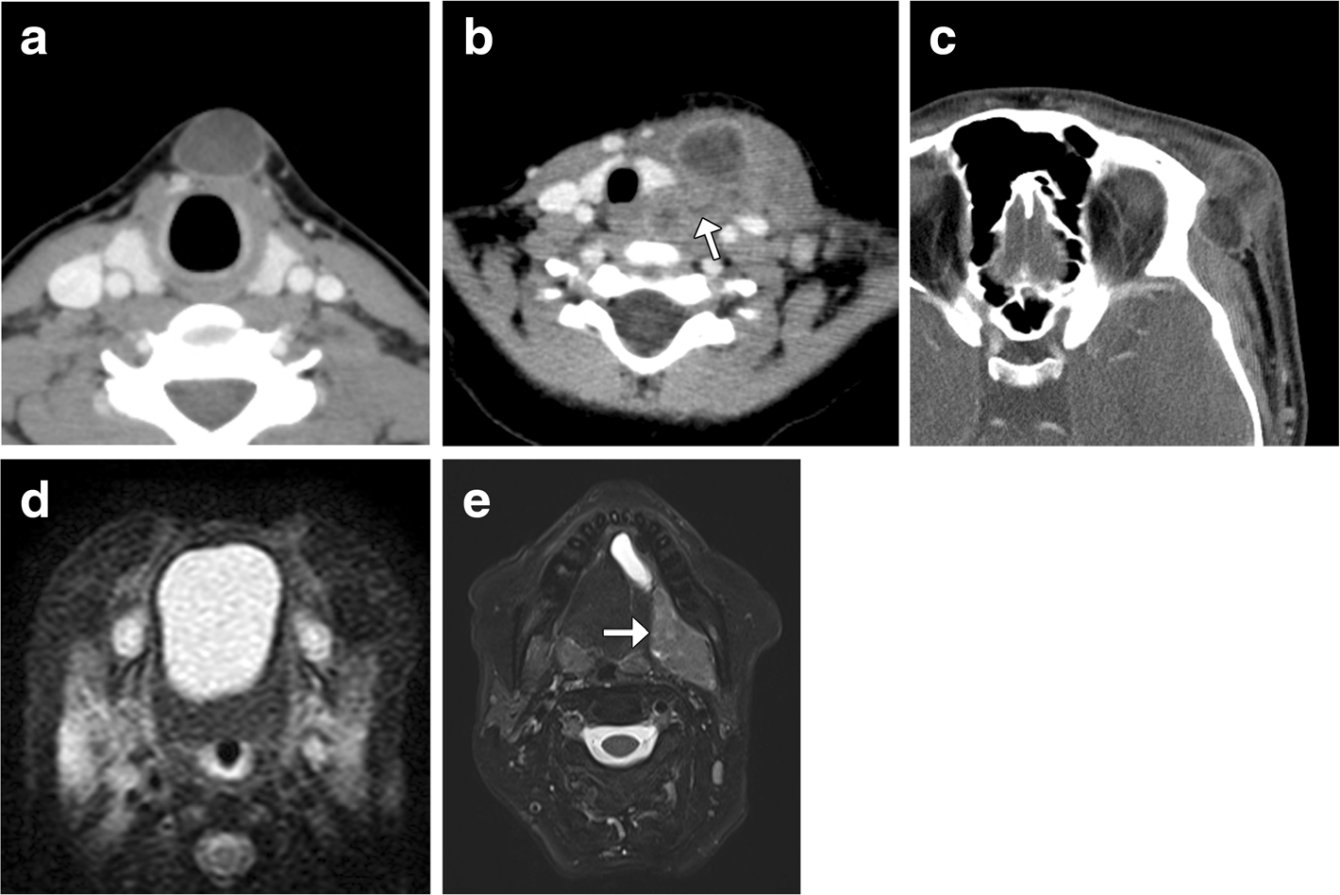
Various tumor-like lesions of the head and neck. Axial CT image (a) shows a midline anterior neck thyroglossal duct cyst. Axial CT image (b) shows an infected left neck branchial cleft cyst with surrounding inflammation and a distended sinus (arrow) that extends towards the hypopharynx. Axial CT image (c) shows a ruptured fat attenuation dermoid in the left lateral periorbital region with surrounding inflammation. Axial fat-suppressed T2-weighted MRI (d) shows a midline oral cavity foregut duplication cyst. Axial fat-suppressed T2-weighted MRI (e) shows a cystadenocarcinoma of the left sublingual region (arrow) associated with a ranula
